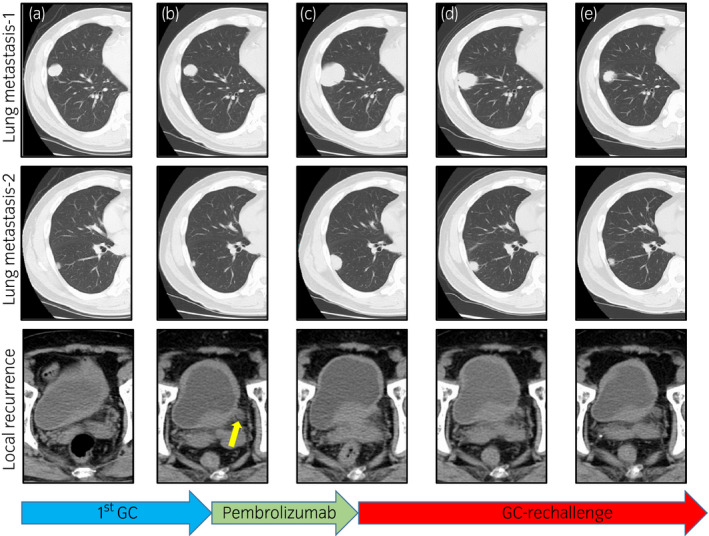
Treatment course after the appearance of lung metastases by CT evaluation. (a) Findings before the start of treatment. Multiple lung metastases and local recurrence are observed (eGFR = 75.7ml/min/1.73m^2^). (b) Findings after two courses of first GC. Multiple lung metastases did not change significantly, but local recurrence progressed. As shown by the yellow arrow, the left hydroureter was newly confirmed (eGFR = 65.4ml/min/1.73m^2^). (c) Findings after four courses of pembrolizumab. Multiple lung metastases were markedly increased (eGFR = 69.7ml/min/1.73m^2^). (d) Findings after one course of re‐challenge GC. Multiple lung metastases were significantly reduced, while local recurrence was unchanged (eGFR = 73.6ml/min/1.73m^2^). (e) Findings after three courses of re‐challenge GC. Multiple lung metastases were further reduced (eGFR = 73.3ml/min/1.73m^2^).
